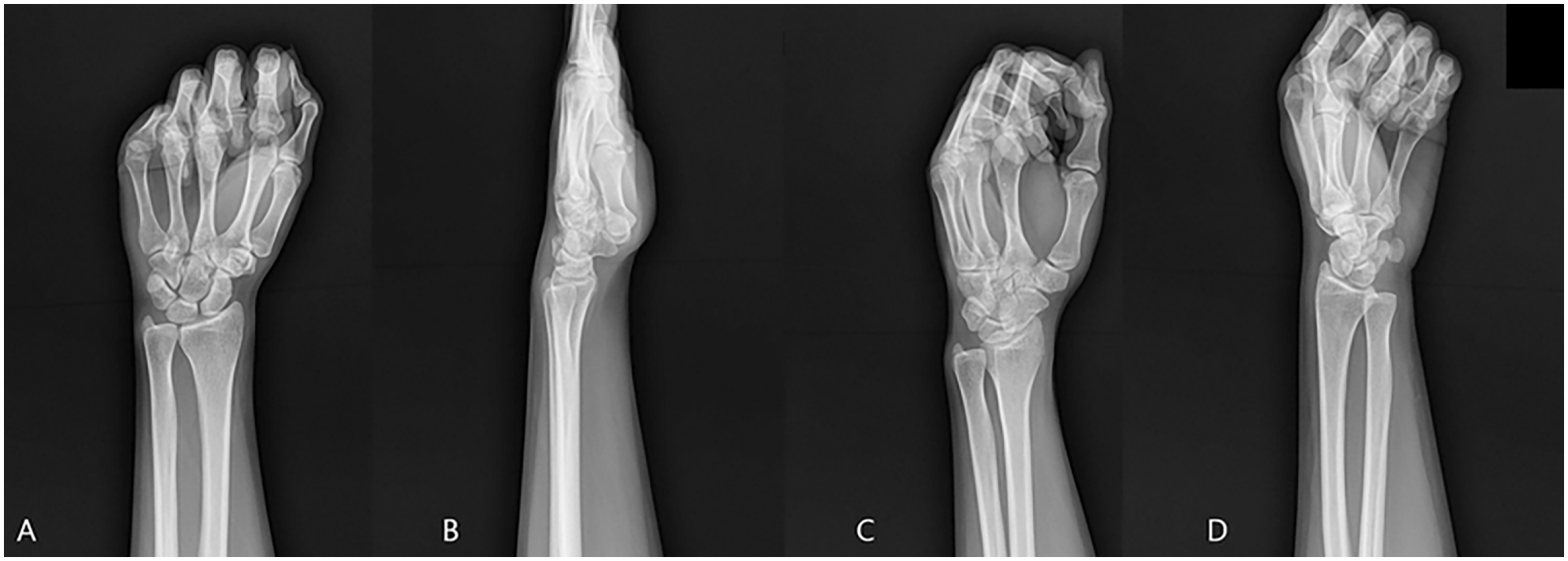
Initial radiographic series of the left wrist (A) Anteroposterior, (B) Lateral, (C) Internal Oblique, (D) External Oblique. No definitive fracture line is observed.
